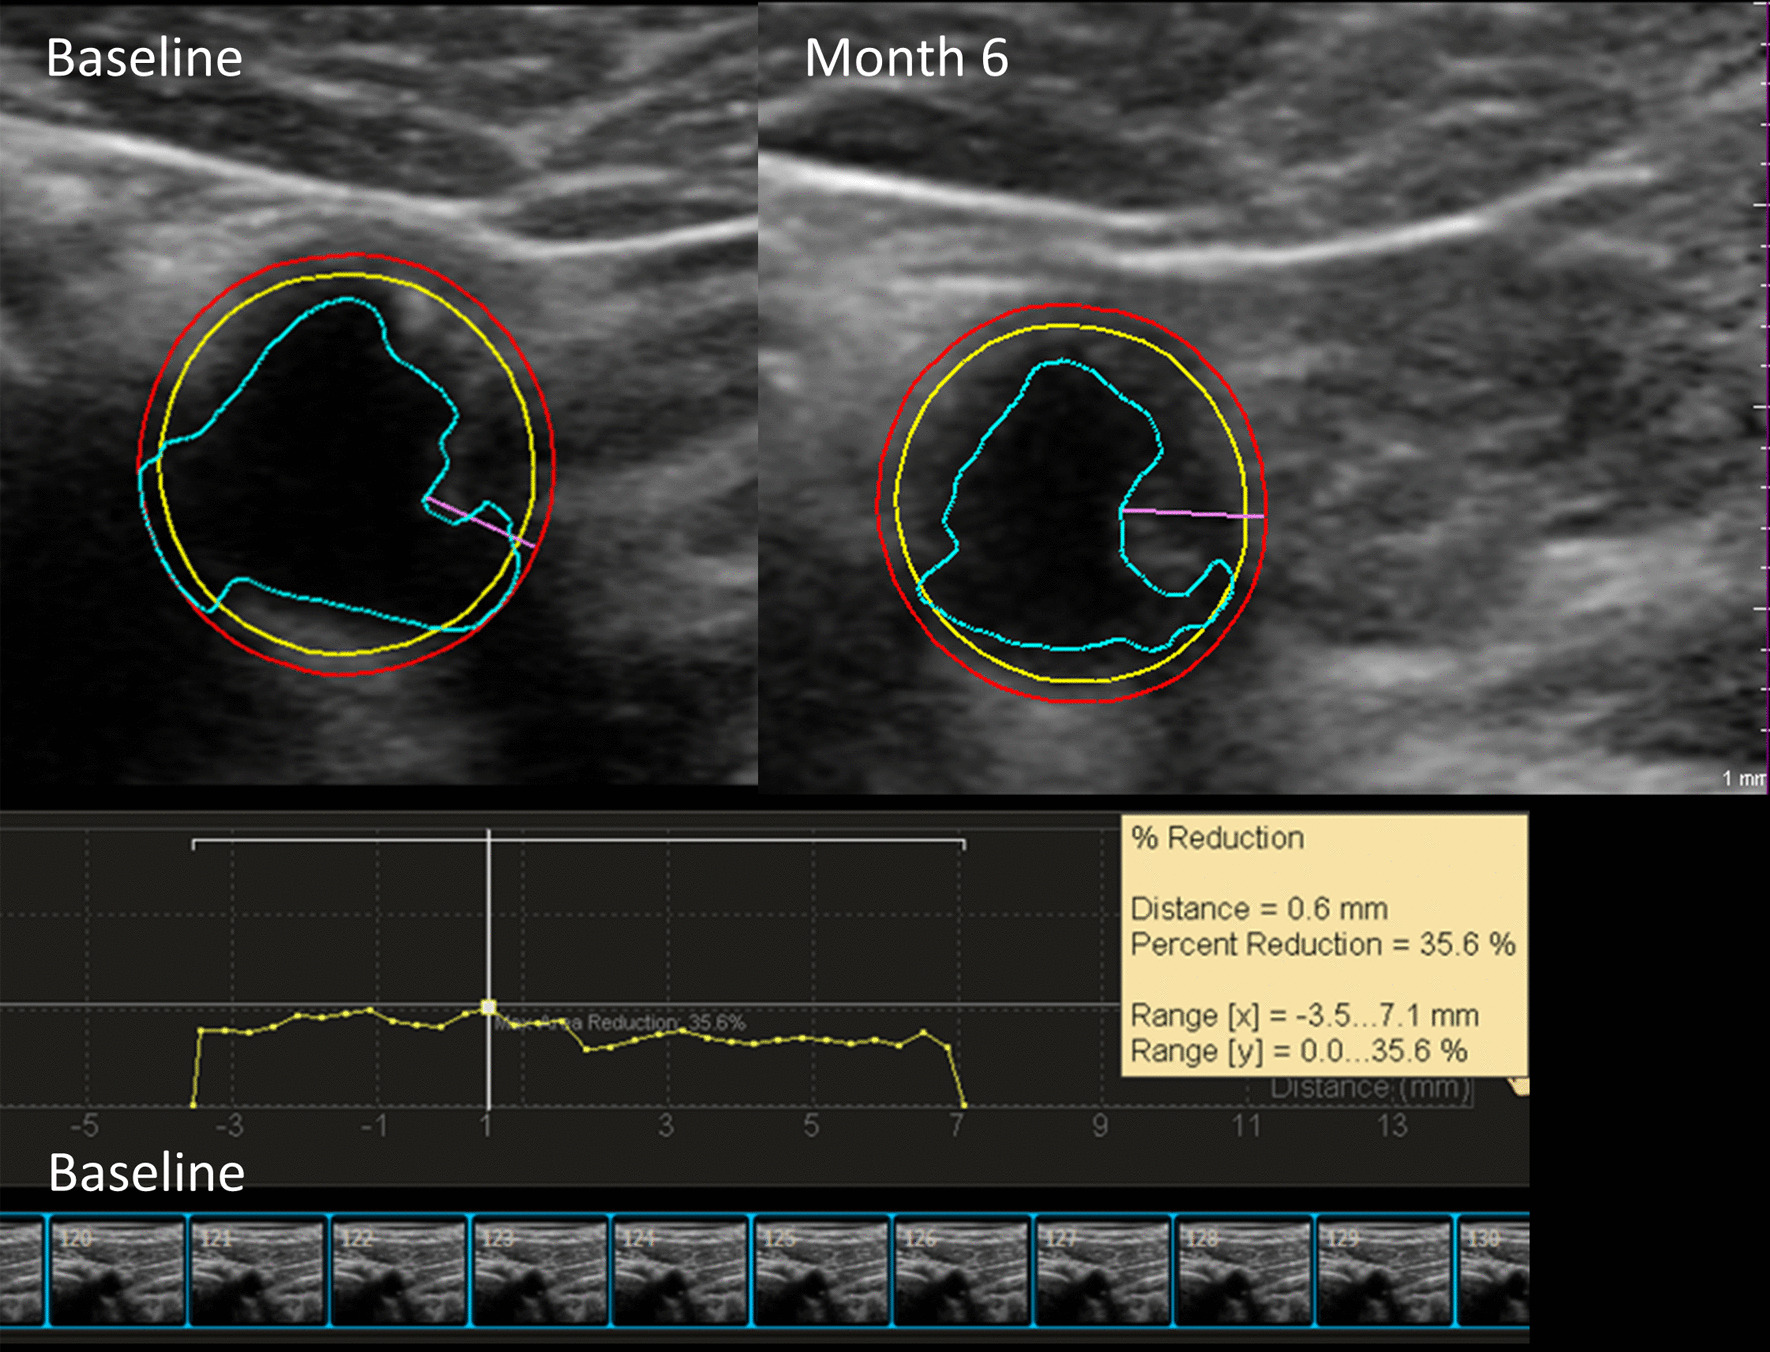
Quantification of carotid arterial plaque acquired by three-dimensional ultrasound. Philips VPQ software was used to assess plaque in 3D. A cross section of the vessel demonstrates a percent lumen reduction (stenosis) of 36% at baseline compared to 47% at month 6
